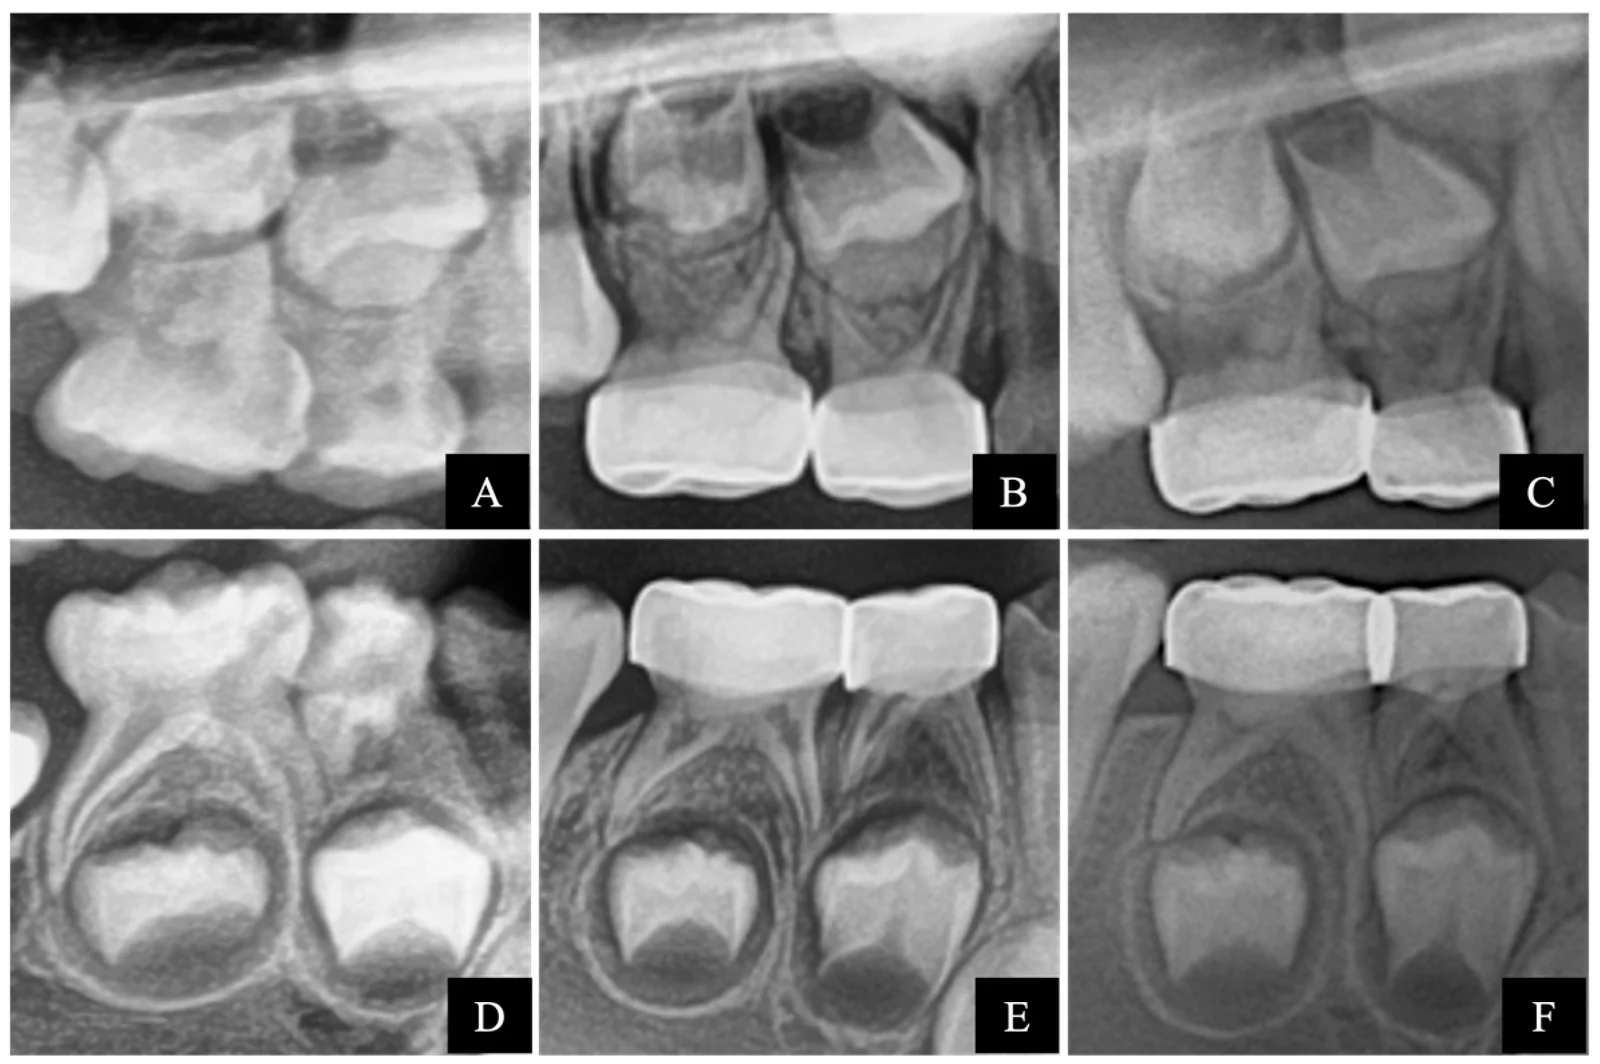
Radiographic evaluation of teeth 54, 55, 84 and 85 in Patient 5. (A–C) Teeth 54 and 55: (A) Preoperative radiograph; (B) 6-month post-treatment radiograph; (C) final follow-up radiograph. (D–F) Teeth 84 and 85: (D) Preoperative radiograph; (E) 6-month post-treatment radiograph; (F) final follow-up radiograph.
